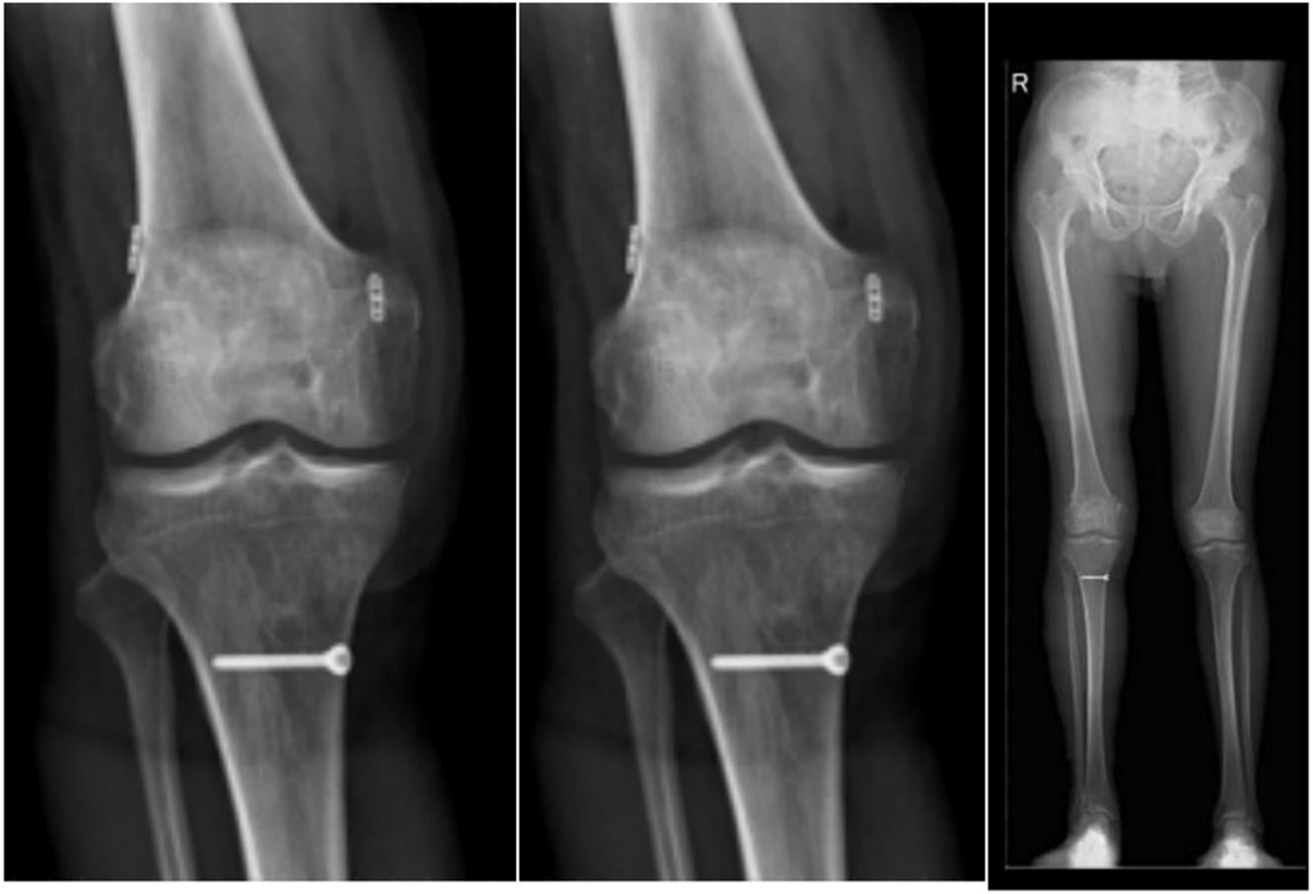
The postoperative X-rays of a 59-year-old man with PLC injury
